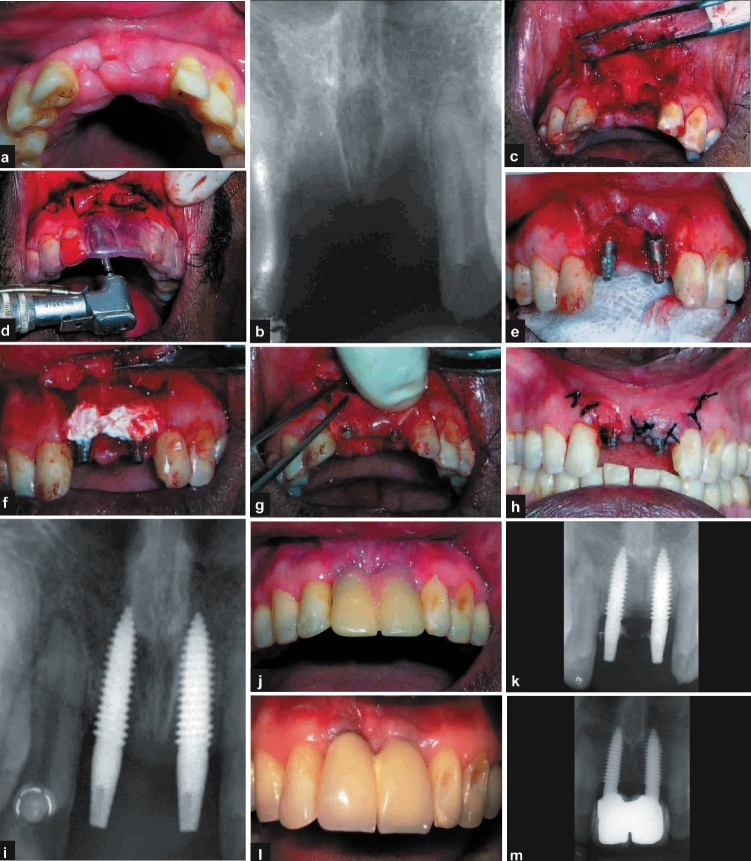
a, b) Pre-operative photograph and radiograph; c- i) operative photographs and radiograph; j-m) post-operative photographs and radiographs
